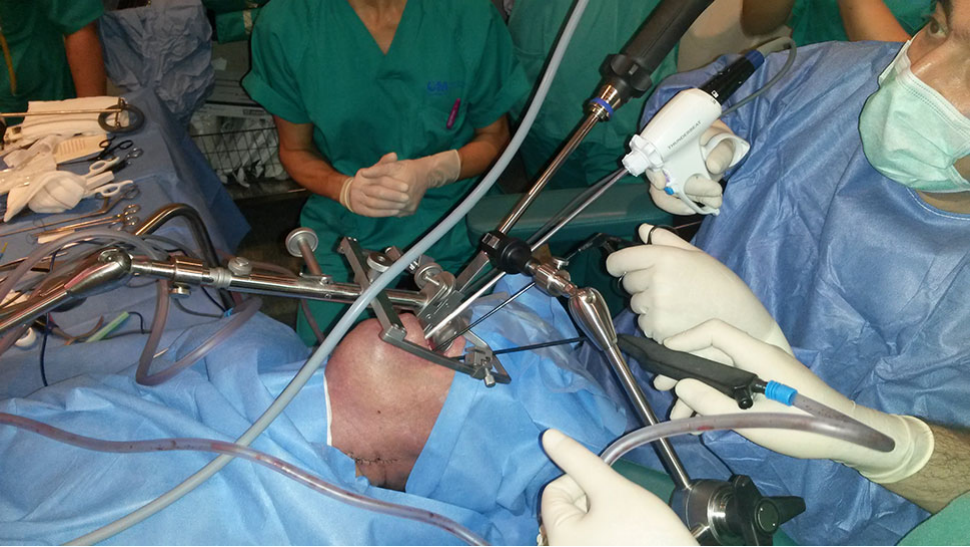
Showing a closer view of TOUSS setup, the use of ultrasonic scalpel for tumoral resection and the assistant by the left side of the surgeon keeping clear the endoscopic vision with a suction cannula
